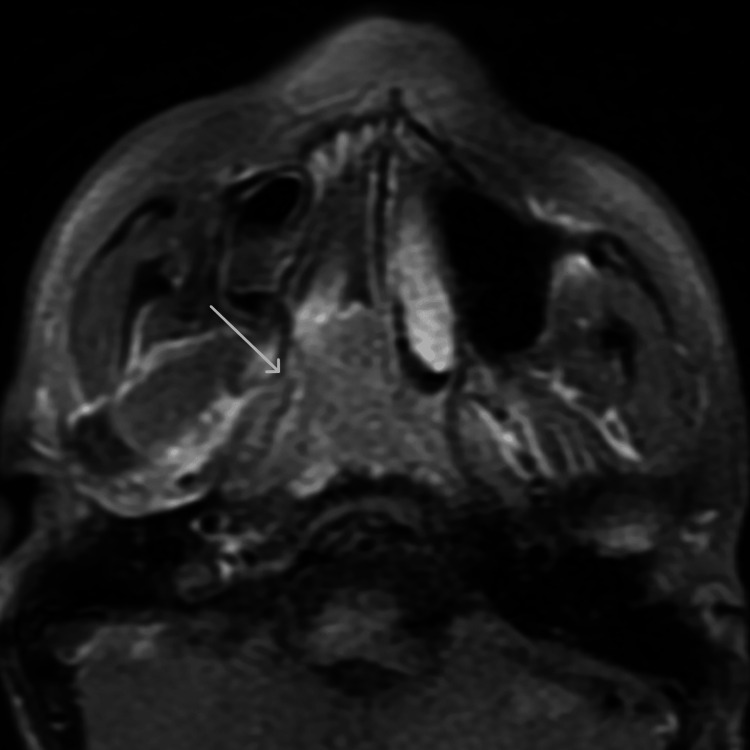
Post-contrast fat-saturated T1-weighted axial image through the skull base A heterogeneously enhancing invasive mass in the roof of nasopharynx with invasion of the right parapharyngeal space and right prestyloid recess is seen as pointed by the arrow.
